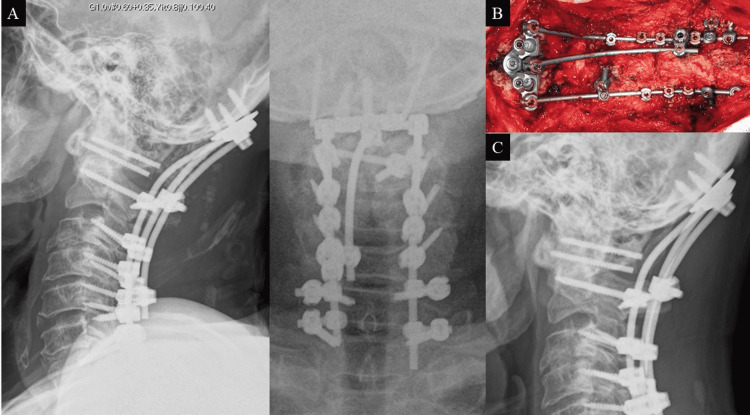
Revision surgery in case 1 (A) X-ray image after Oc-T1 fusion with triple rod connection. (B) Intraoperative photograph obtained before bone grafting demonstrates the triple rod connection. (C) X-ray image obtained 12 months after revision surgery shows bony fusion without implant failure.
